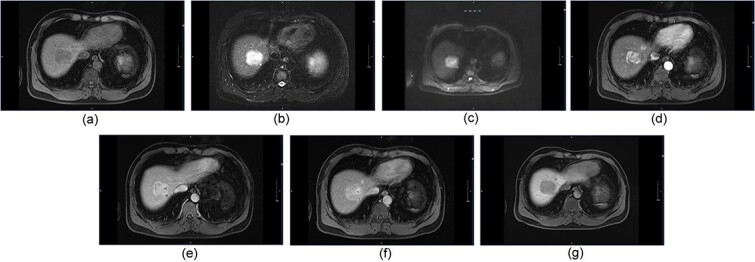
(a) On T1-weighted imaging (T1WI), the lesions exhibit low signal intensity, similar to that on non-contrast computed tomography (CT). On T2-weighted imaging (T2WI). (b) and diffusion-weighted imaging (DWI). (c) the lesions show heterogeneous high signal intensity relative to the liver parenchyma. (d-g) on dynamic contrast-enhanced MRI scans, the largest lesion shows immediate peripheral ring-like enhancement with progressive continuous enhancement with a central enhancing scar. Other smaller ones show ring-like enhancement, with gradual centripetal enhancement on the portal venous and delayed phases.
